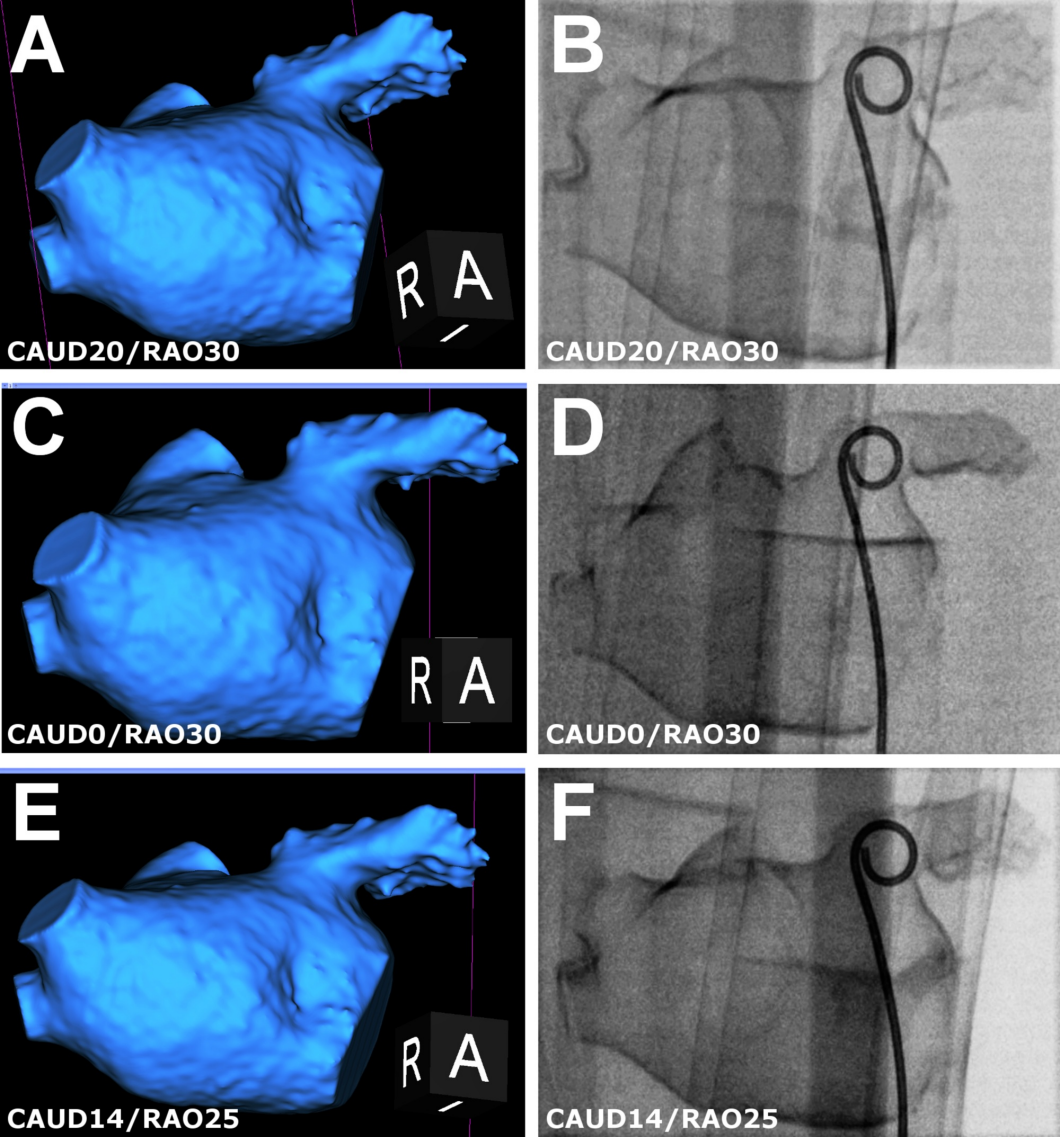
Personalized views in 3D CT and model fluoroscopy Optimal fluoroscopic views estimated on 3D CT (left) are successfully replicated on 3D printed LAA model fluoroscopy (right). The pigtail catheter in the LAA is for calibration (right). (A, B) Standard view (CAUD20/RAO30); (C, D) optimized for maximum length (CRAN0/RAO30); (E, F) optimized for maximum diameter (CAUD14/RAO25). 3D, three-dimensional; LAA, left atrial appendage; RAO, right anterior oblique; LAO, left anterior oblique; CAUD, caudal; CRAN, cranial.
